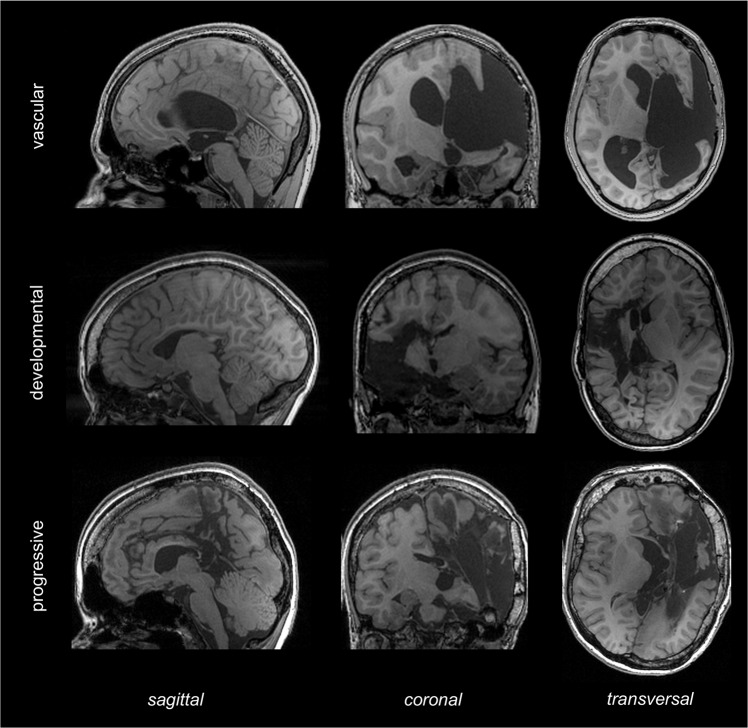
T1-weighted sequence of three exemplary patients after hemispherotomy.
